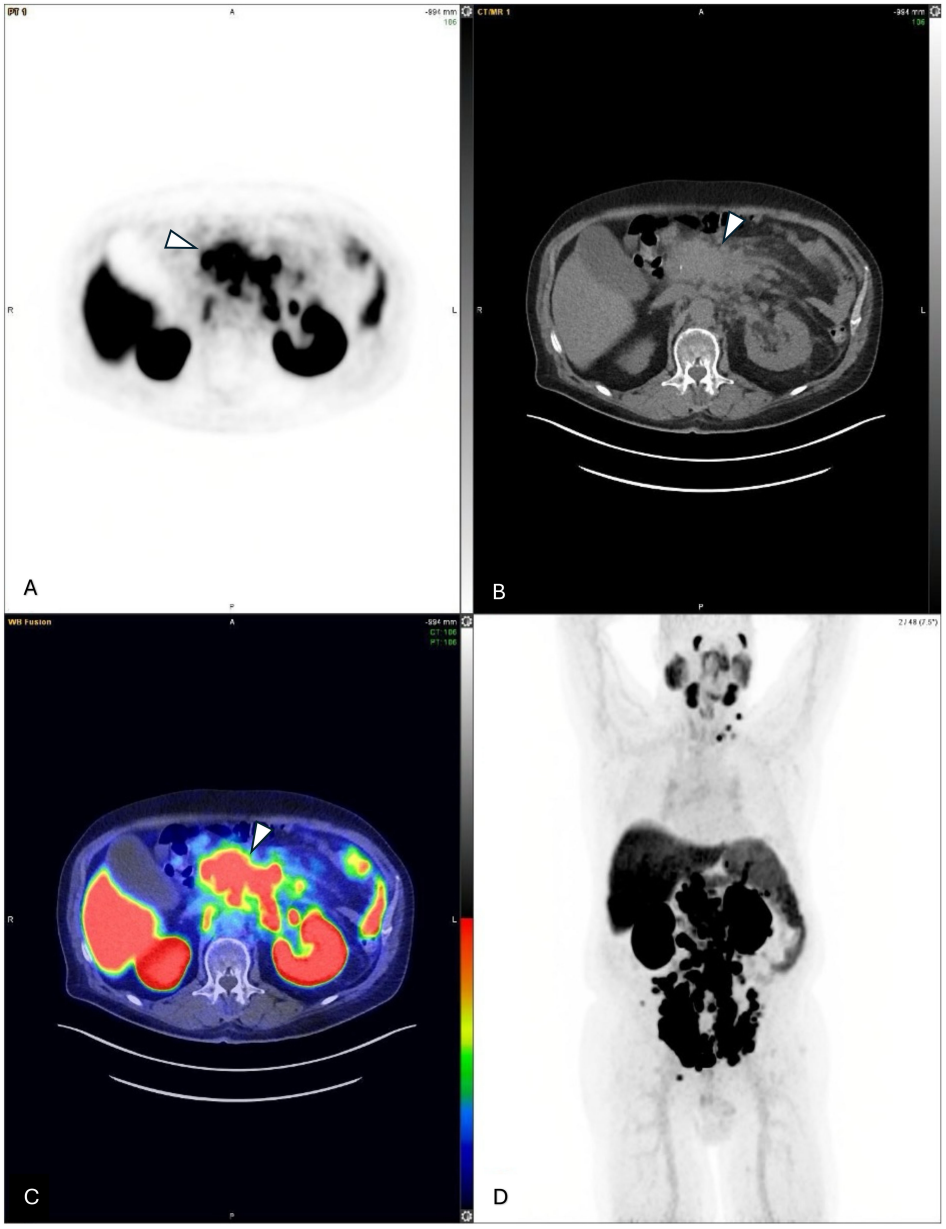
(A–C) Axial views of multiple metastatic lymph nodes adjacent to the pancreas from a pre-therapy ^68^Ga-PSMA-PET/CT scan, indicated by arrowheads (molecular imaging PSMA score, 3). (D) A pre-therapy ^68^Ga-PSMA PET/CT maximum intensity projection (MIP) image showing multiple PSMA-avid metastatic lymph nodes both below and above the diaphragm.
